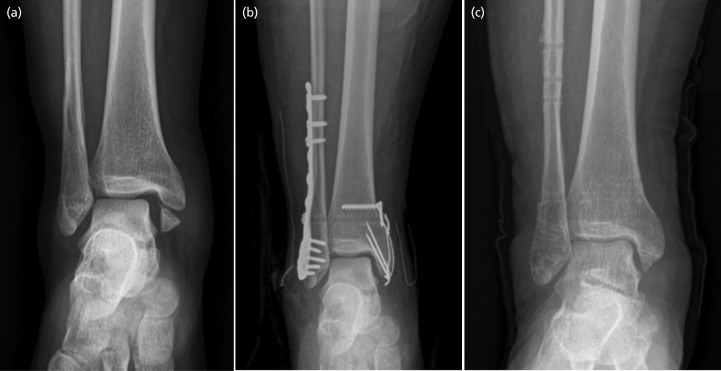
Dynamic fixation of syndesmosis. (a) Pre-operative anteroposterior radiograph of right ankle showing pronation external rotation-type ankle fracture. (b) The syndesmosis was fixed with TightRope. (c) At the one-year follow-up, all implants were removed; radiograph showing complete bone union and well-maintained ankle mortise.
